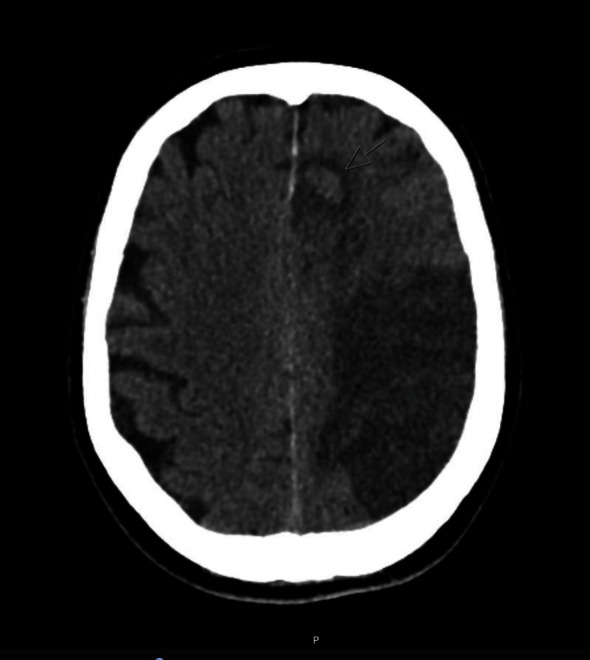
Noncontrast CT brain performed on day 3, revealing haemorrhage in the ACA territory.
